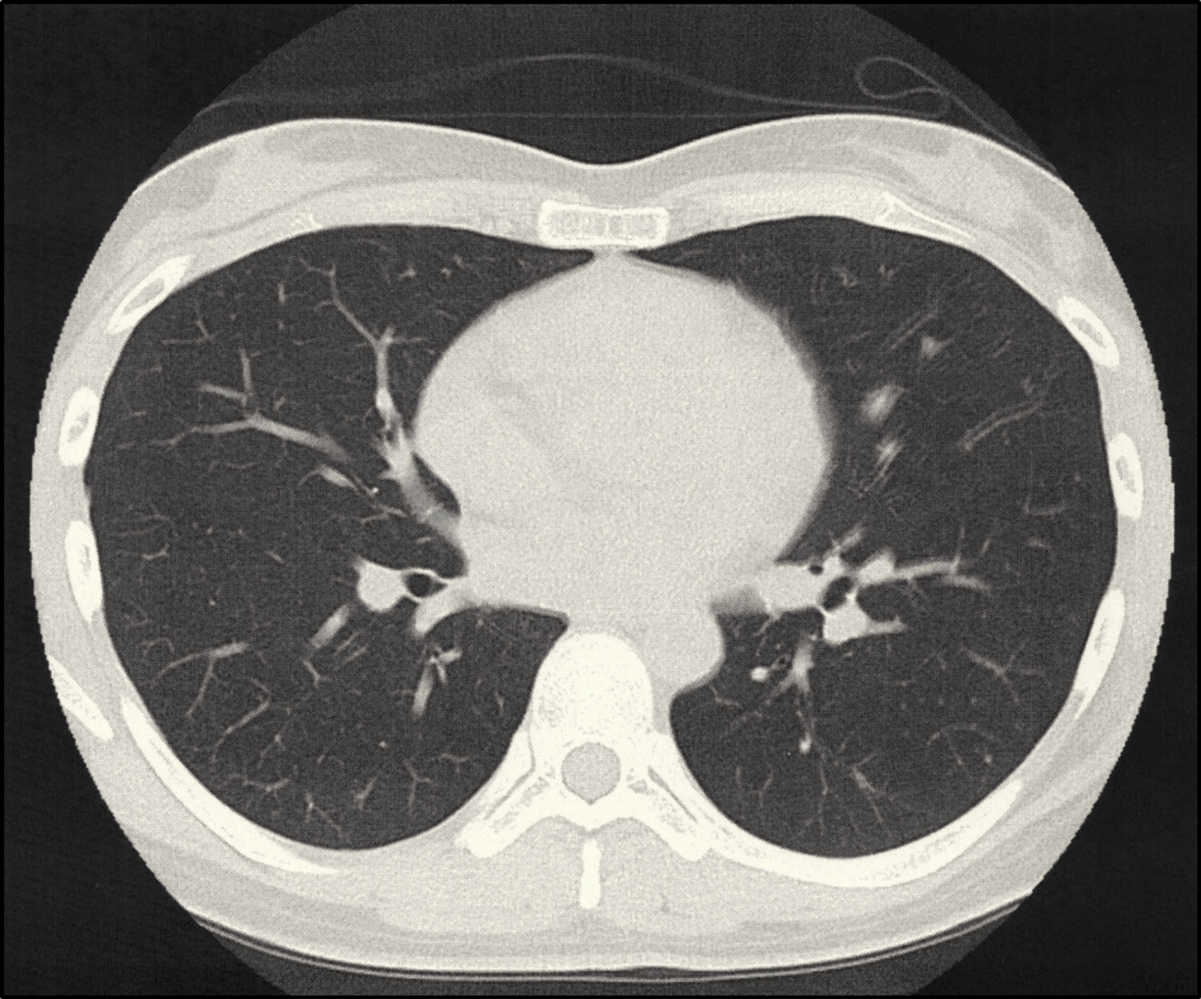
CT scan of the chest The chest CT scan revealed no evidence of pneumonia.
